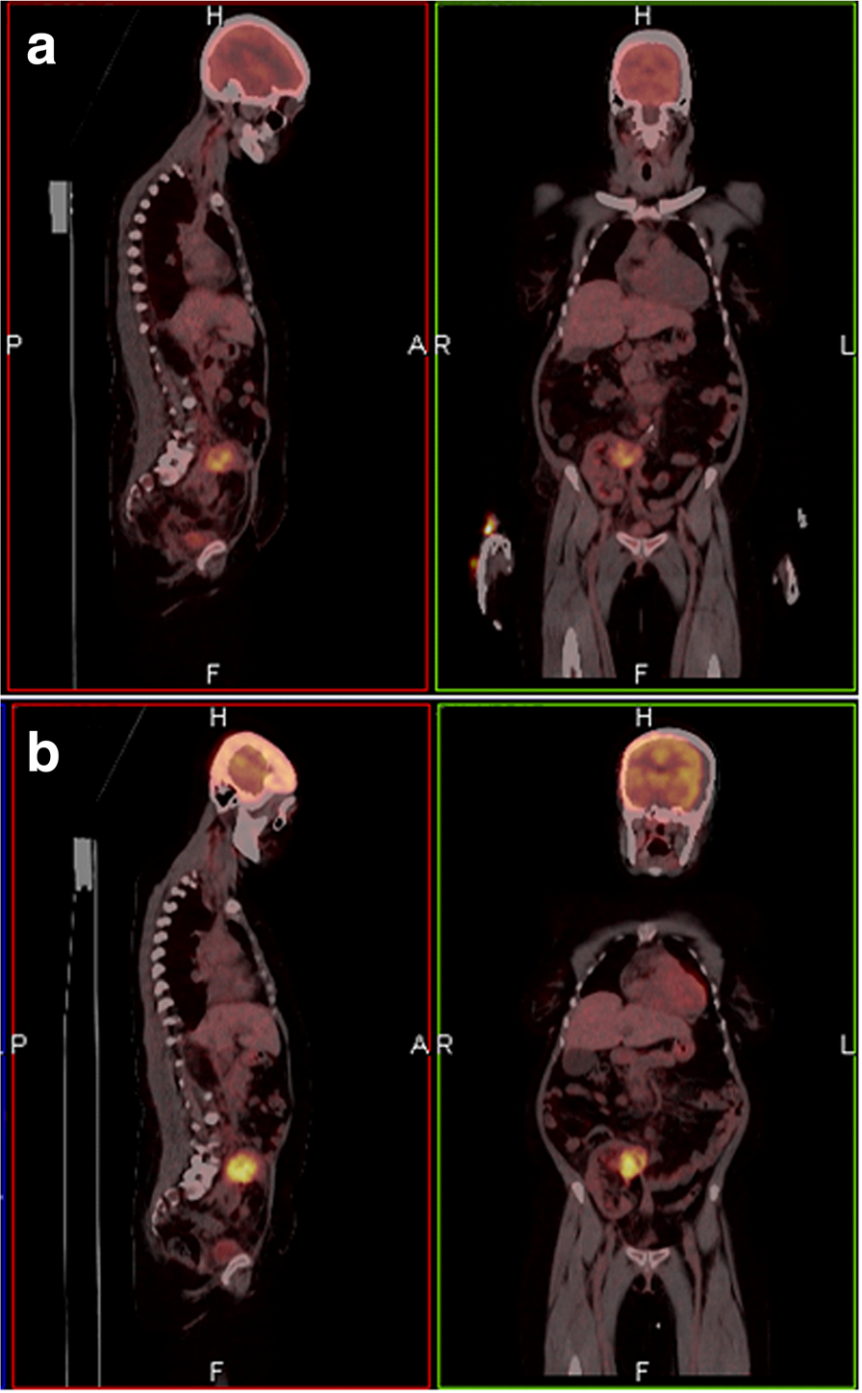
FDG-PET/CT scan demonstrating a metabolically active lesion in the transplanted kidney (panel a), and exacerbation on follow-up scan (panel b)
